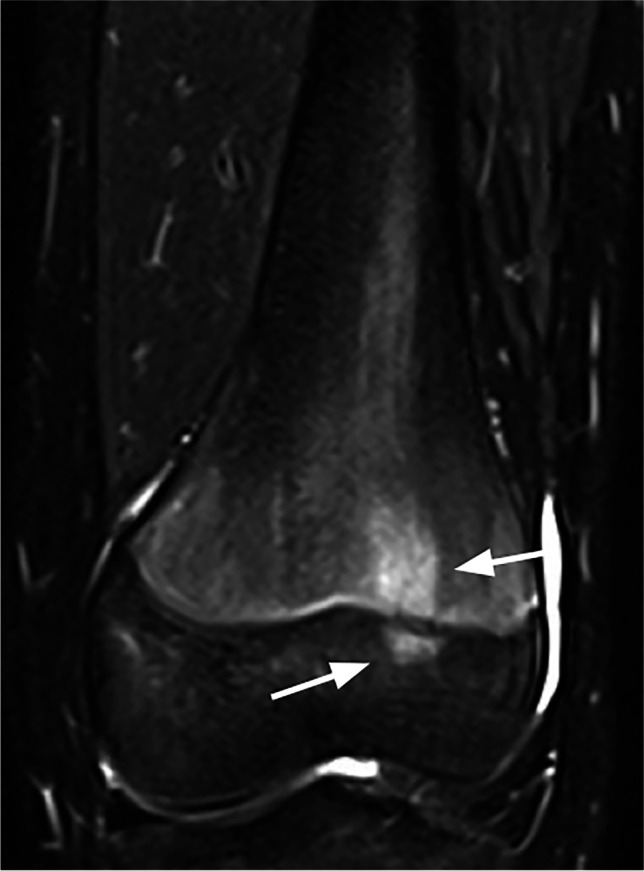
Coronal water-only Dixon T2-weighted magnetic resonance image in a 14-year-old girl with an area of high signal intensity with the same width on both sides of the physis scored as focal periphyseal edema (FOPE) (*arrows*)
